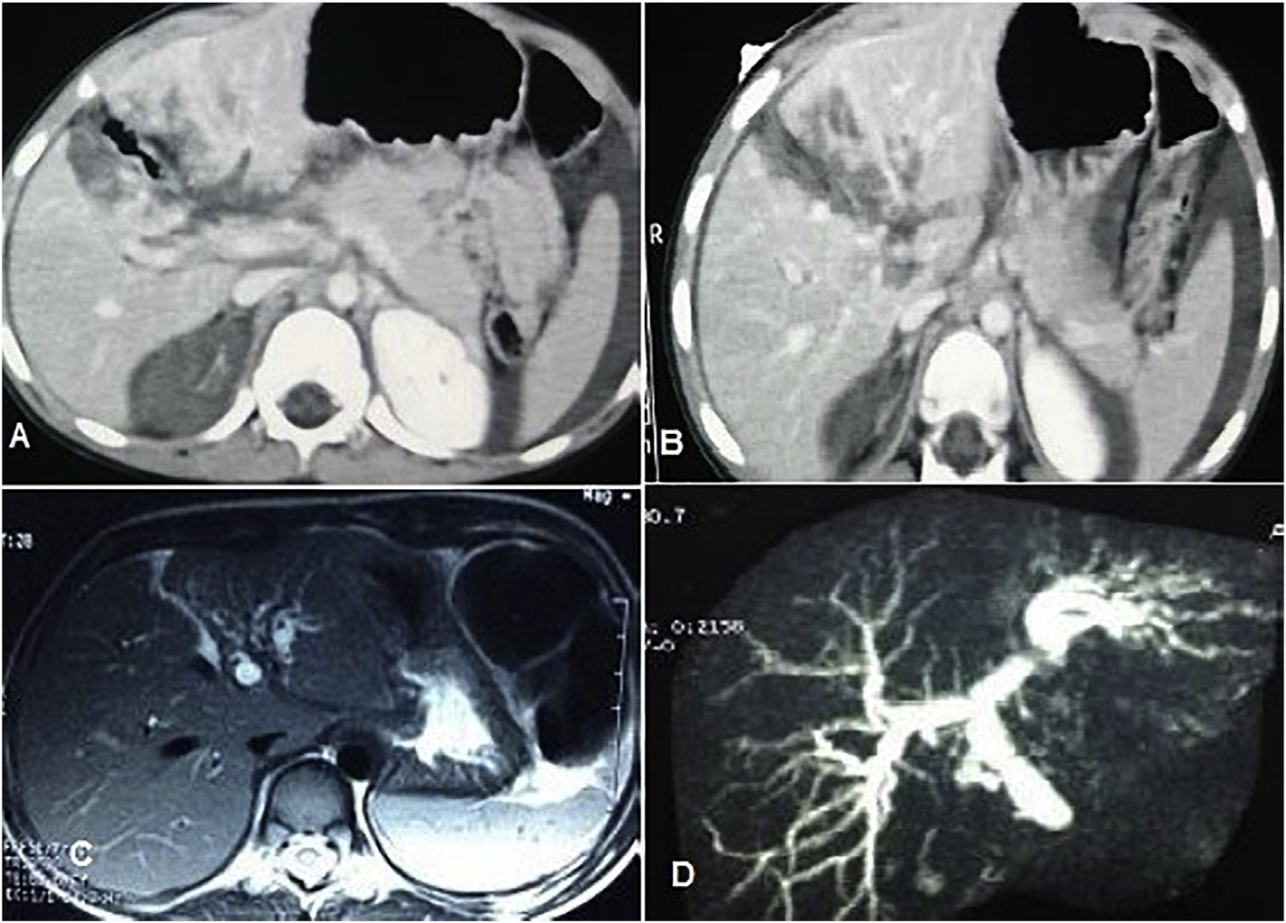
A, B) CT shows grade IV trauma, large complex tear about 9 cm involving the central portion of the liver segment III, IV, VIII and it branches laterally to involve segment V, VI and medially to involve caudate lobe. C, D) MRCP after 5 months of the trauma showed strictures at left hepatic duct and CHD with subsequent dilated biliary radicles.
